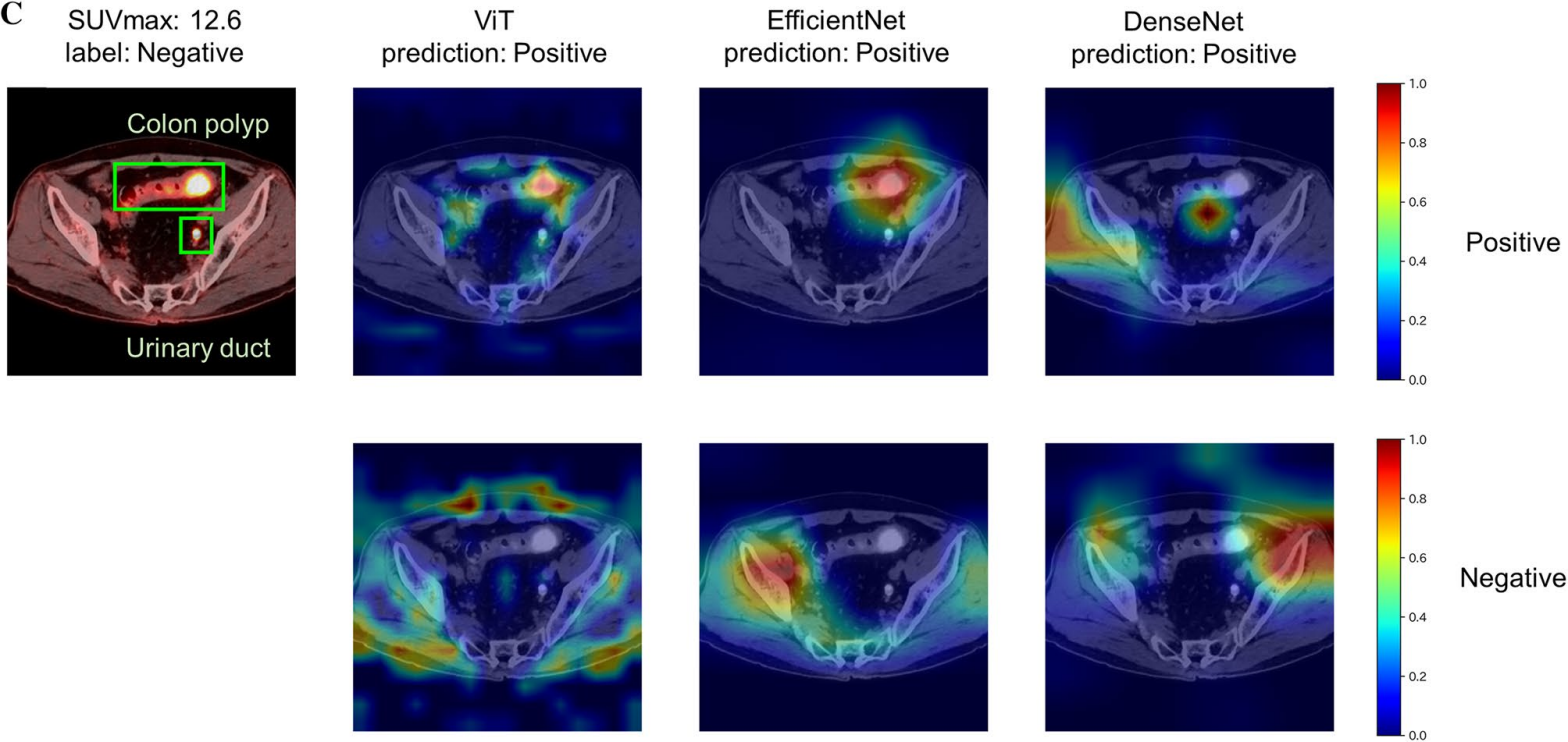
Predictions and Grad-CAMs of ViT, EfficientNet, and DenseNet models on sample test images. The yellow bounding boxes indicate malignant lesions and the green bounding boxes present benign lesions. The top rows of the Grad-CAMs show important areas for a “positive” prediction, and the bottom rows show areas for a “negative” prediction. *SUVmax* Maximum standardized uptake value, *ViT* Vision Transformer, *Grad-CAM* Gradient-weighted class activation mapping.
